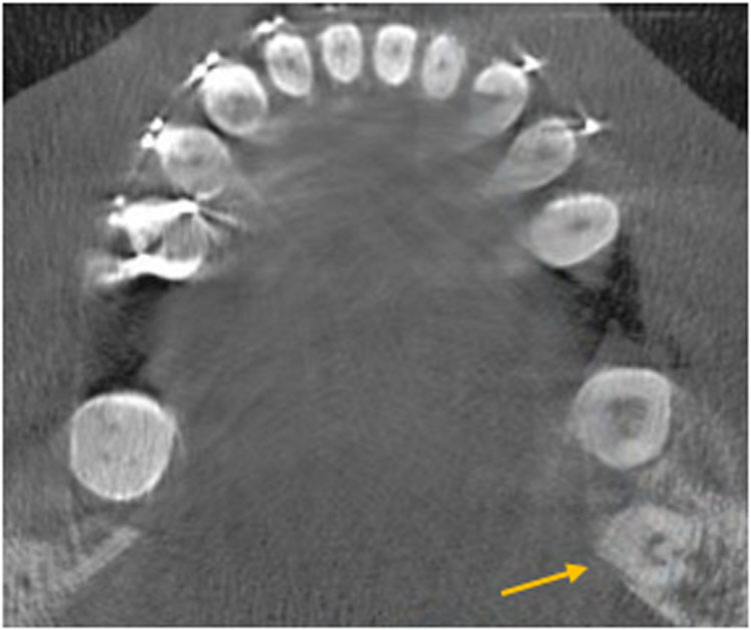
Axial CBCT view of a representative mandibular third molar with a C‐shaped canal. CBCT, cone‐beam computed tomography.
